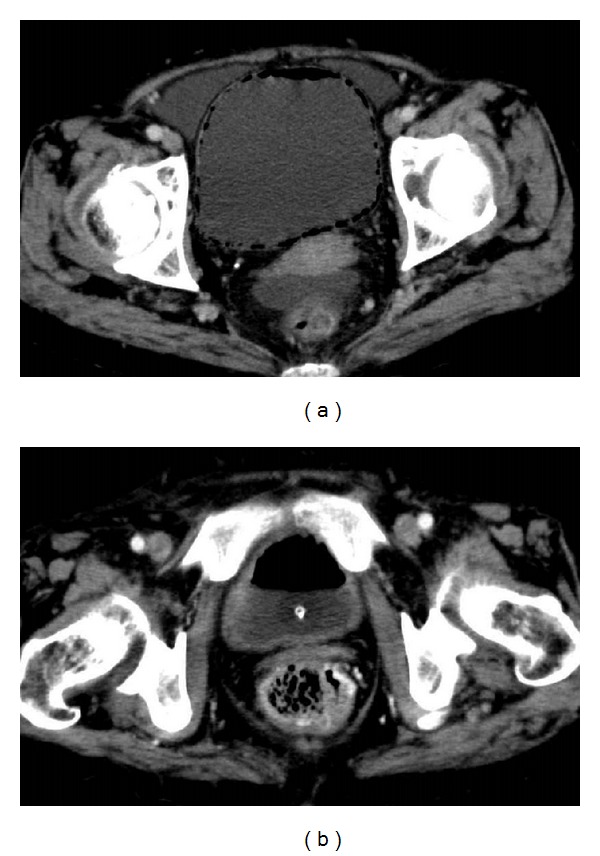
CT findings of the pelvis. (a) CT showed diffuse air within the bladder wall. (b) CT after treatment showed disappearance of air within the bladder wall and normally observed air in the bladder lumen during placement of Foley catheter.
